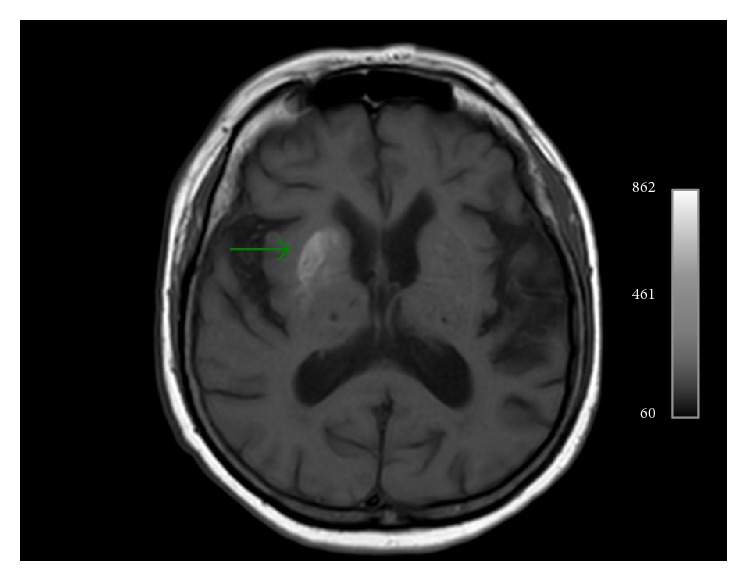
Striking increase of T1-weighted signal intensity within right striatum one month after the onset of symptoms. Patient was asymptomatic when this MR study was performed.
